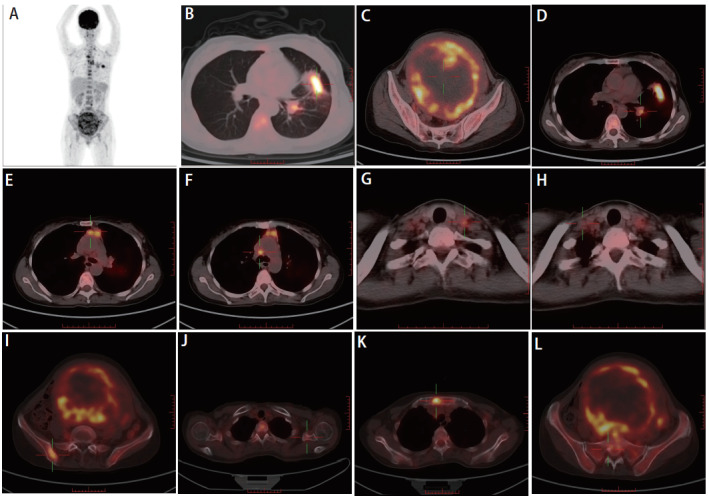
患者病灶的PET/CT影像学资料。A：全景图；B：左肺上叶舌段肿块；C：子宫肿块；D：左肺门区淋巴结转移；E：前纵隔区淋巴结转移；F：纵隔4R区淋巴结转移；G：左腋窝淋巴结转移；H：右锁骨区淋巴结转移；I：右侧髂血管淋巴结转移；J：左侧肩胛骨转移；K：胸骨转移；L：骶骨转移。
